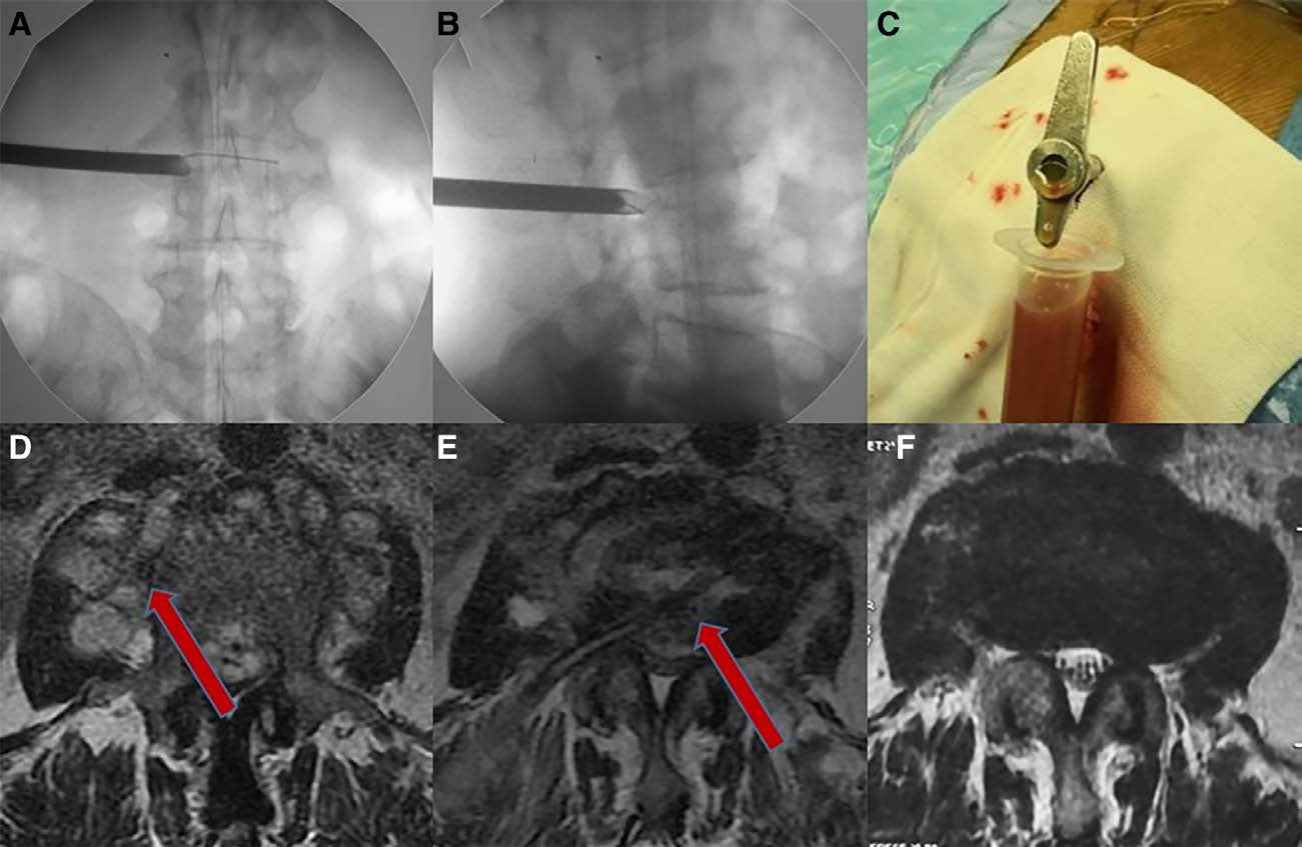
(A) Foraminal endoscopy-assisted lesion removal and cannulation. (B) The spontaneous outflow of tuberculous pus during the operation indicates that the pressure of the abscess in the intervertebral space is too high. (C) The L5/S1 space and the right psoas major muscle abscess formed. The arrows indicate that the psoas abscess comes from the perfusion dissemination of the intervertebral space. (D) MRI during postural drainage treatment, the drainage tube indicated by the arrow is in the intervertebral space and fixed in place. (E) Eighteen months after the operation, the MRI shows that the lesion is still, the abscess disappeared. MRI = magnetic resonance imaging.
